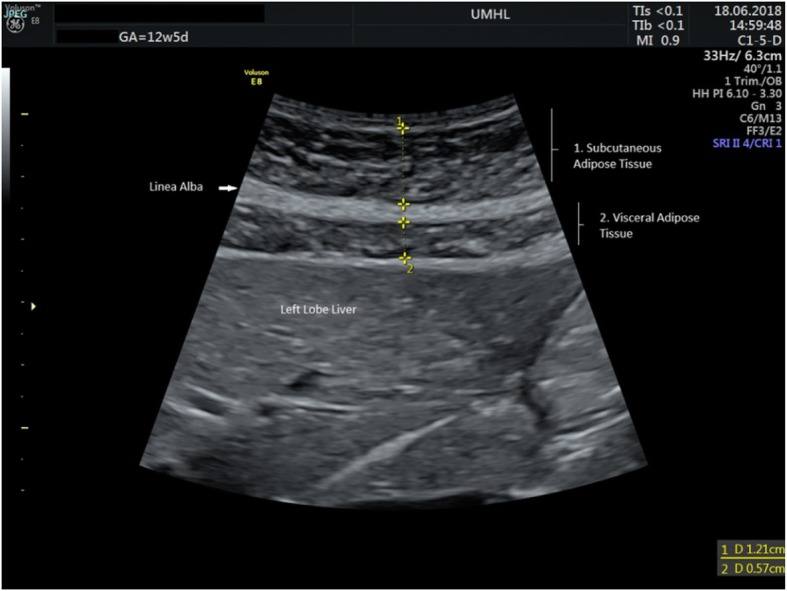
Example ultrasound screenshot image at correct position for measurement of SAT (1) and VAT (2)
